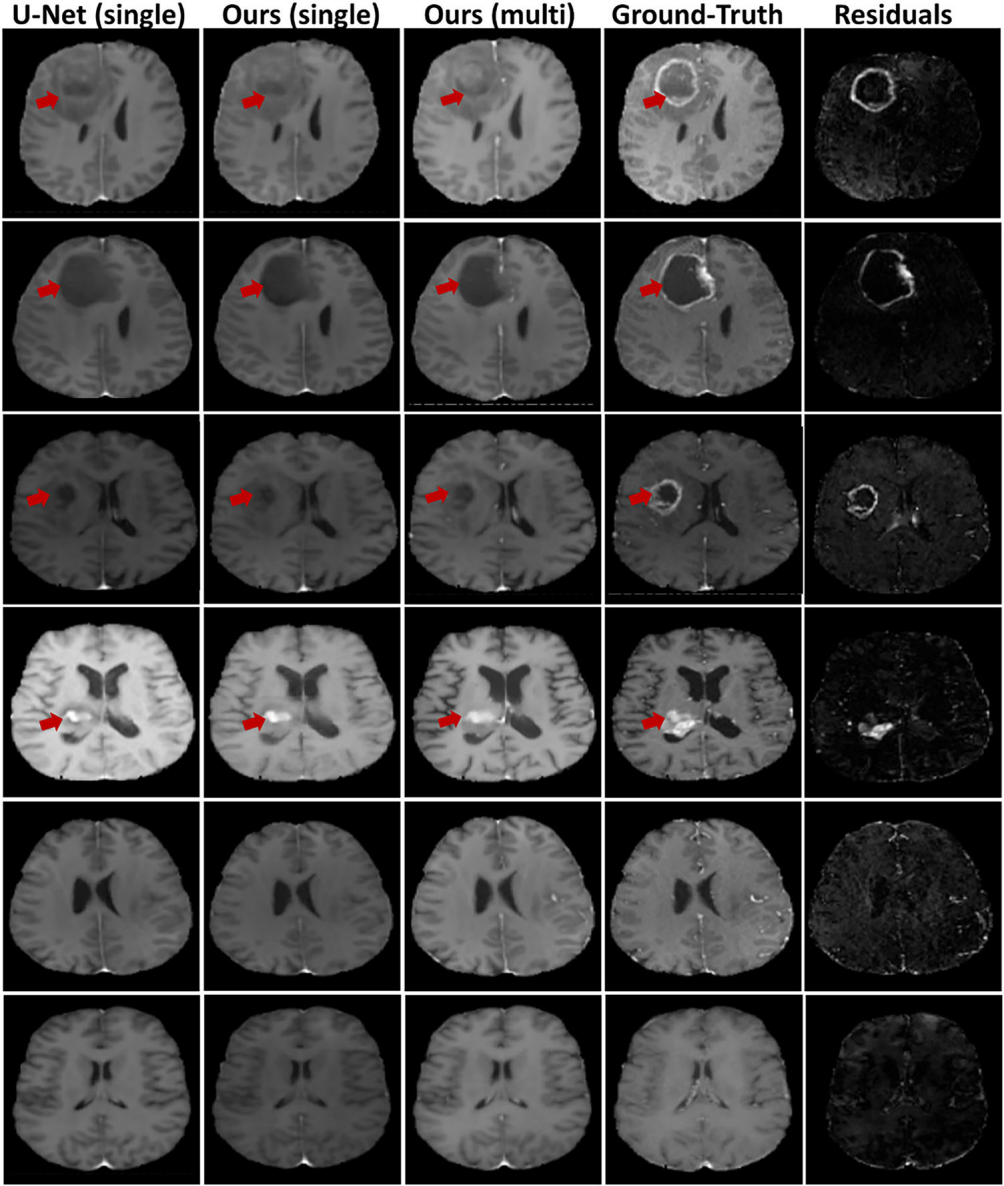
Visual assessment of our proposed DD‐Res U‐Net model for contrast‐enhanced T1 MRI synthesis and a 3D U‐Net baseline model for 6 patients on the test set. From left to right: contrast‐enhanced T1 synthetic image using only T1 as input (DD‐Res U‐Net); contrast‐enhanced T1 synthetic image using only T1 as input (DD‐Res U‐Net); contrast‐enhanced T1 synthetic image using T1, T2, and FLAIR as input (DD‐Res U‐Net); ground‐truth image; and residuals (absolute difference between synthetic and ground‐truth). Rows show results for different patients.
